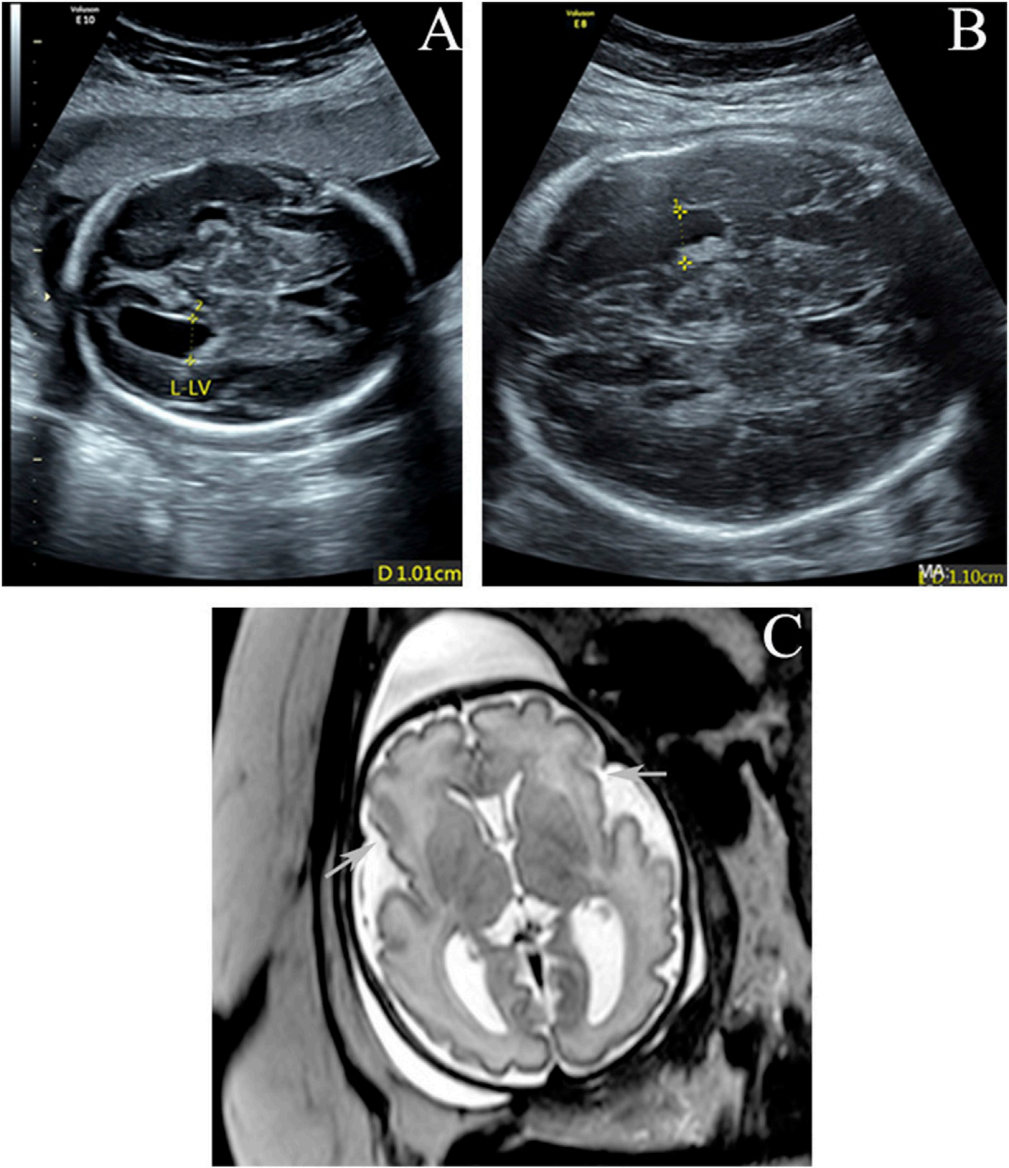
(A,B) Enlarged left lateral ventricle (10.1 and 11 mm) at 25 and 31 gestational weeks. (C) Axial T2-weighted imaging at 32 weeks of gestation shows poor bilateral and frontal operculum formation and shallow bilateral lateral fissures (arrows). The left ventricle is slightly wider.
